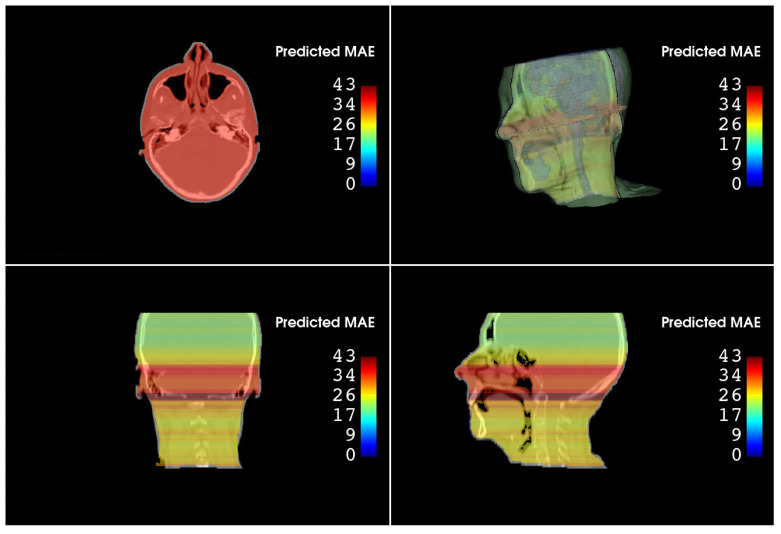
Exemplary $sCT_{CBCT}$ overlaid with its $pMAE_{volume}$. In addition to the 2D views (axial, sagittal, and coronal planes), the 3D representation is also shown.
